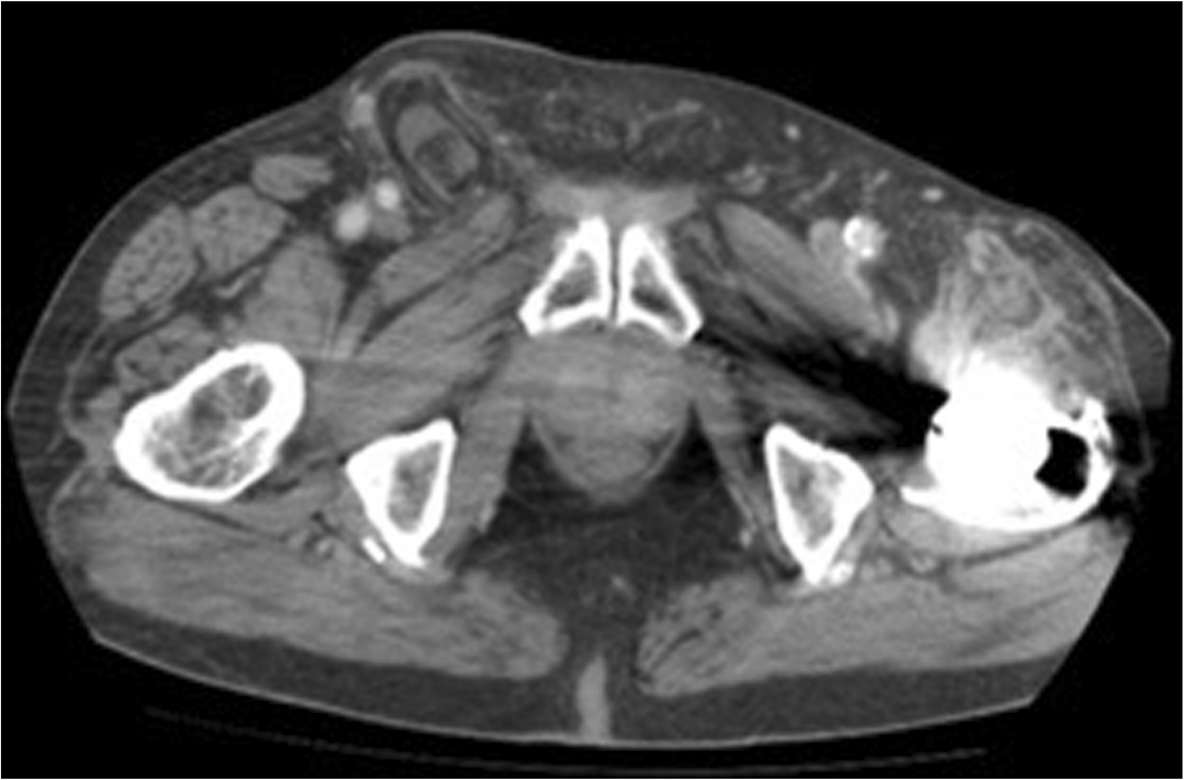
Computed tomography anterior-posterior view of described Amyand hernia
